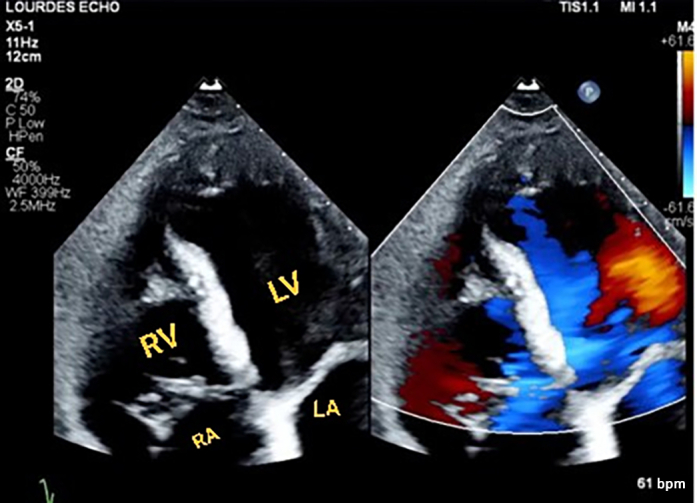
Follow-Up Transesophageal Echocardiogram A follow-up transesophageal echocardiogram recorded 1 month after the procedure revealed no residual shunt. Abbreviations as in Figures 2 and 6.
